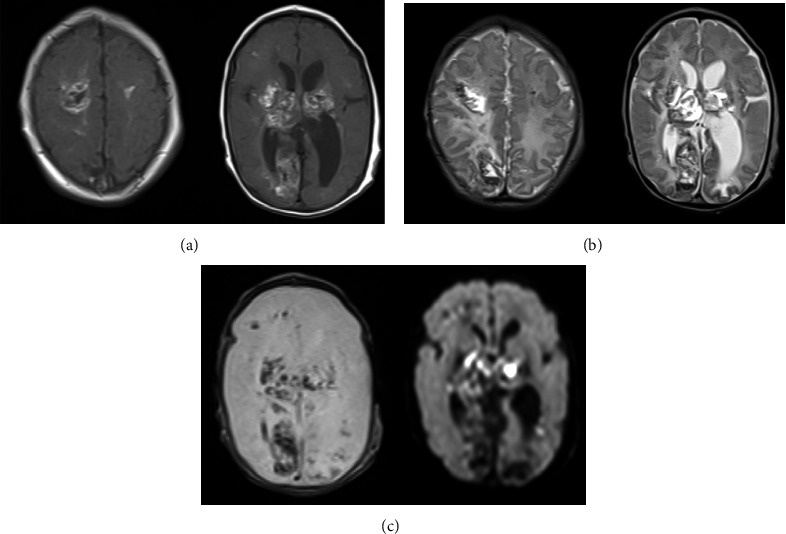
Brain MRI, multiple hemorrhagic infarcts with intraventricular perforation. (a): T1-weighted images, (b): T2-weighted images, and (c): T2^*∗*^ and DWI images.
